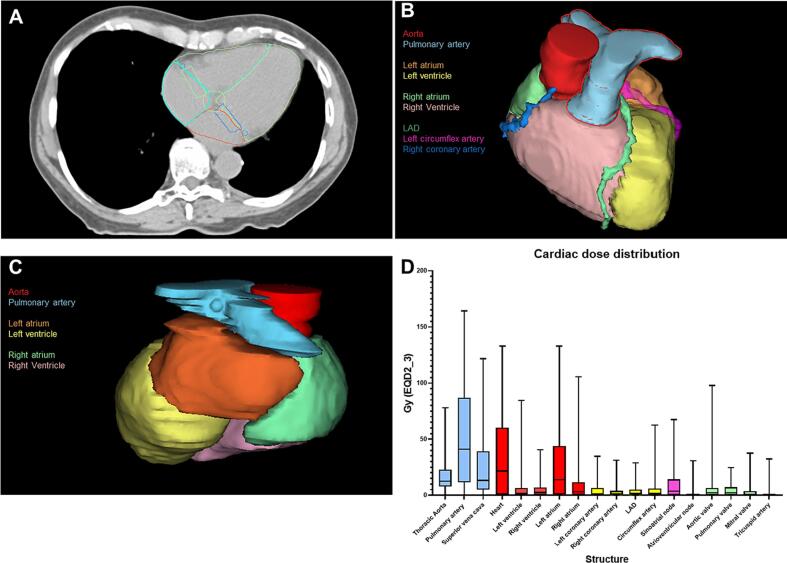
(A-D): (A) auto-segmentation of the heart and 17 heart substructures on planning CT, (B) 3D visualization of the auto-segmented heart substructures (anterior), (C) posterior view of 3D visualization of the auto-segmented heart substructures, (D) cardiac dose distribution (D0.1 cc in EQD2_3), showing large vessels highlighted (blue), cardiac chambers (red), coronary arteries (yellow), pacemaker regions (purple) and heart valves (green).
